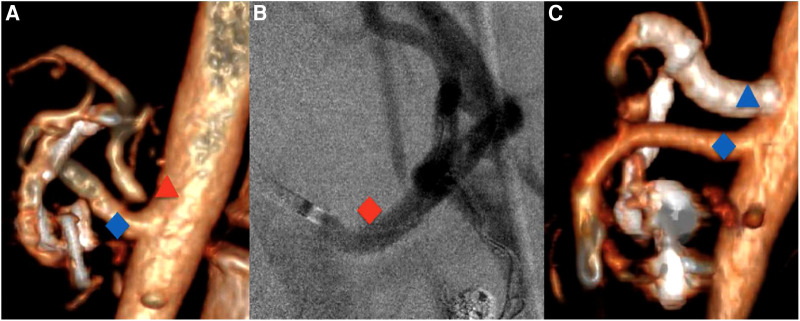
(A) Preoperative 3D computed tomography with the CT stenosis (red triangle) and the patent SMA (blue diamond). (B) Perioperative CT arteriography after stenting (red diamond). (C) Postoperative 3D computed tomography with the patent CT stents (blue triangle) and SMA (blue diamond). CT, celiac trunk; SMA, superior mesenteric artery.
